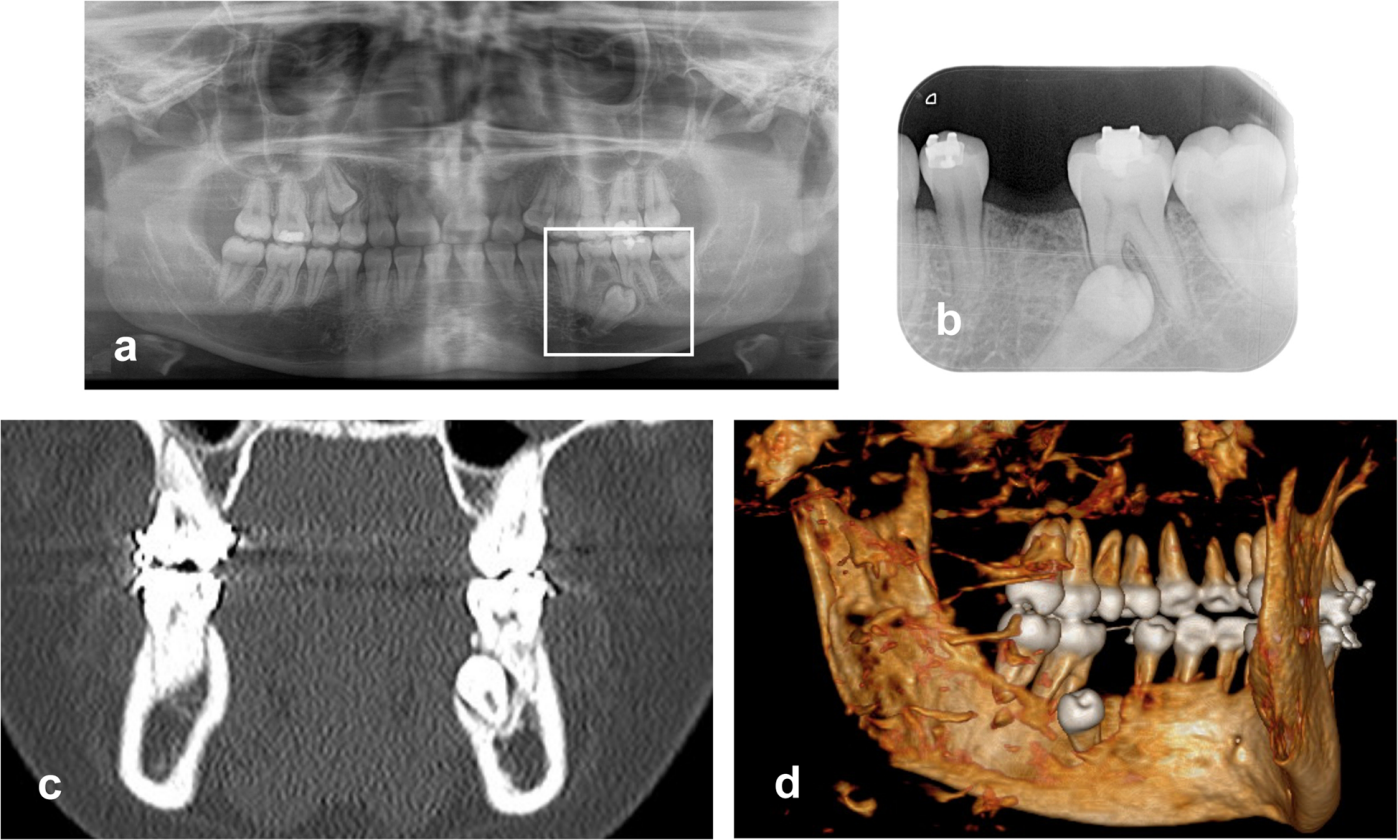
Pre-intervention. a Orthopantomography; b Periapical x-ray 4 months after extraction of tooth 75; c and d CT showing the position of tooth 35 (lingual view)
